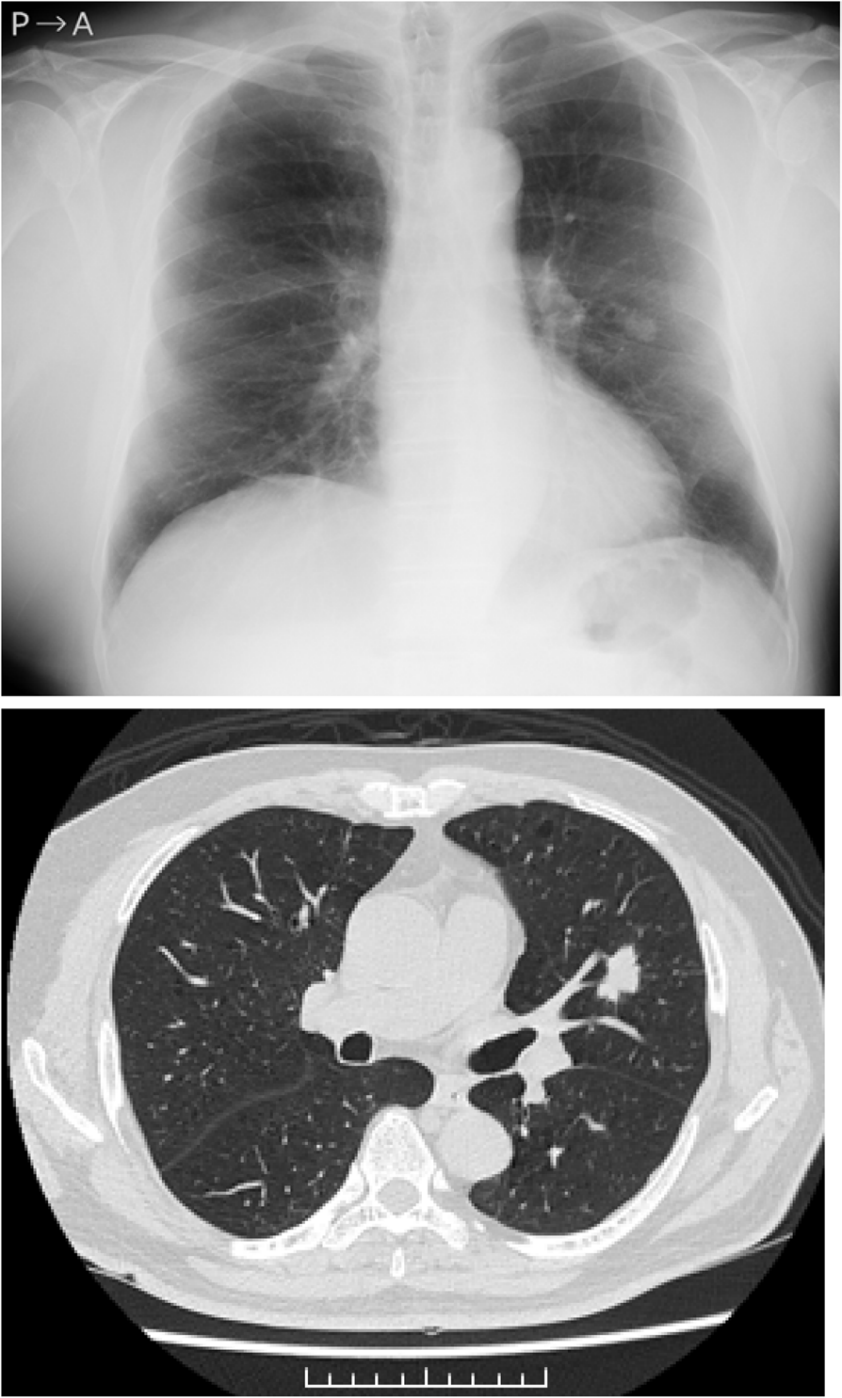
Chest X-ray and computed tomographic images. a Chest X-ray film showing a tumor shadow in the left middle field. b Chest computed tomography showing a 25 × 13 mm nodule in the left upper lobe
